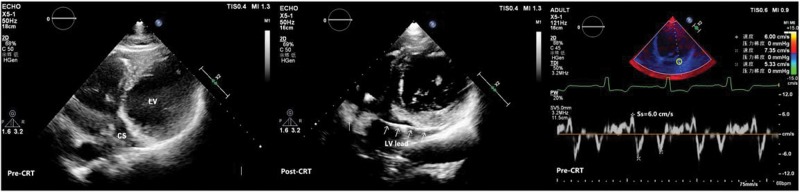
Example of myocardial velocity curve via tissue Doppler imaging. The sample was obtained from the left ventricular lead-implanted segment (yellow circle); the Y-axis represents myocardial velocity (cm/s), and the X-axis represents time; Ss is the positive peak on the curve.
